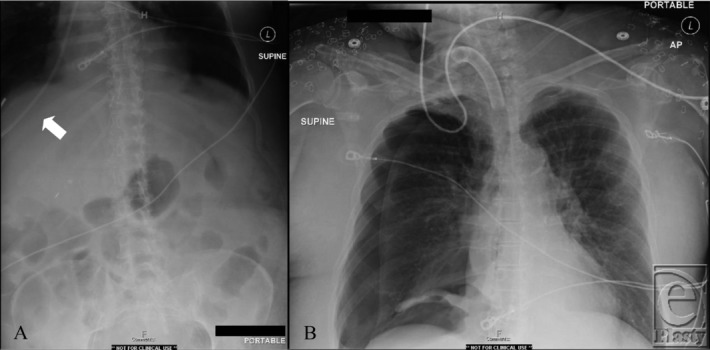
Plain films (*a*) of the abdomen following small bowel feeding tube placement and (*b*) of the chest after immediate removal of the tube.
